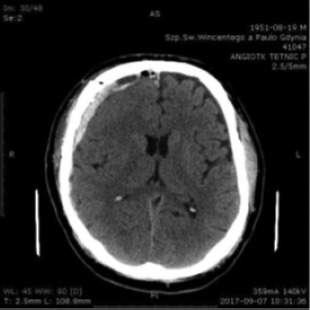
Head CT scan, September 7, 2017
